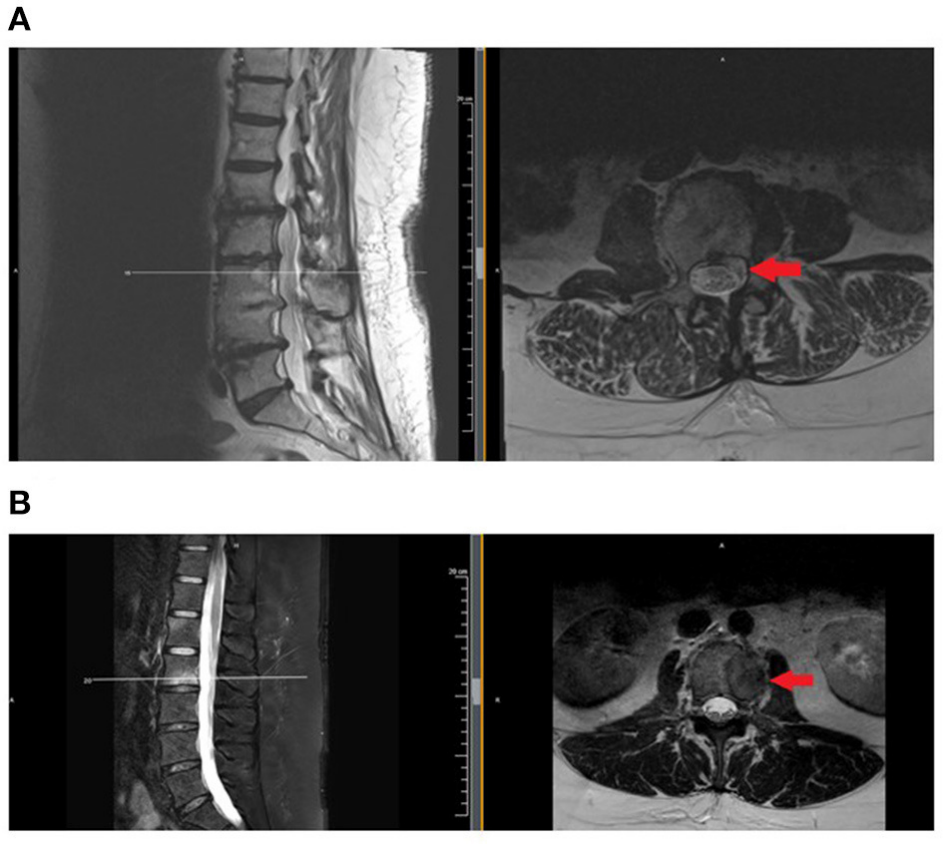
Examples of patients with vertebral metastatic disease, involving the epidural space (A) and confined to osseous structures. (B) Injections should be avoided or administered with extreme caution when disease involves the epidural space. MRI is the test of choice to evaluate for epidural disease. MRI, magnetic resonance imaging.
